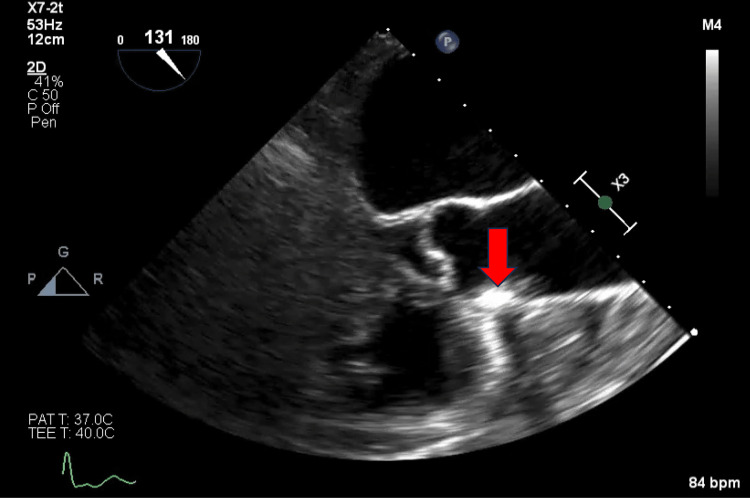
Transesophageal echocardiogram showing mobile echo density (indicated by a red arrow) distal to the aortic valve on the wall of the ascending thoracic aorta
